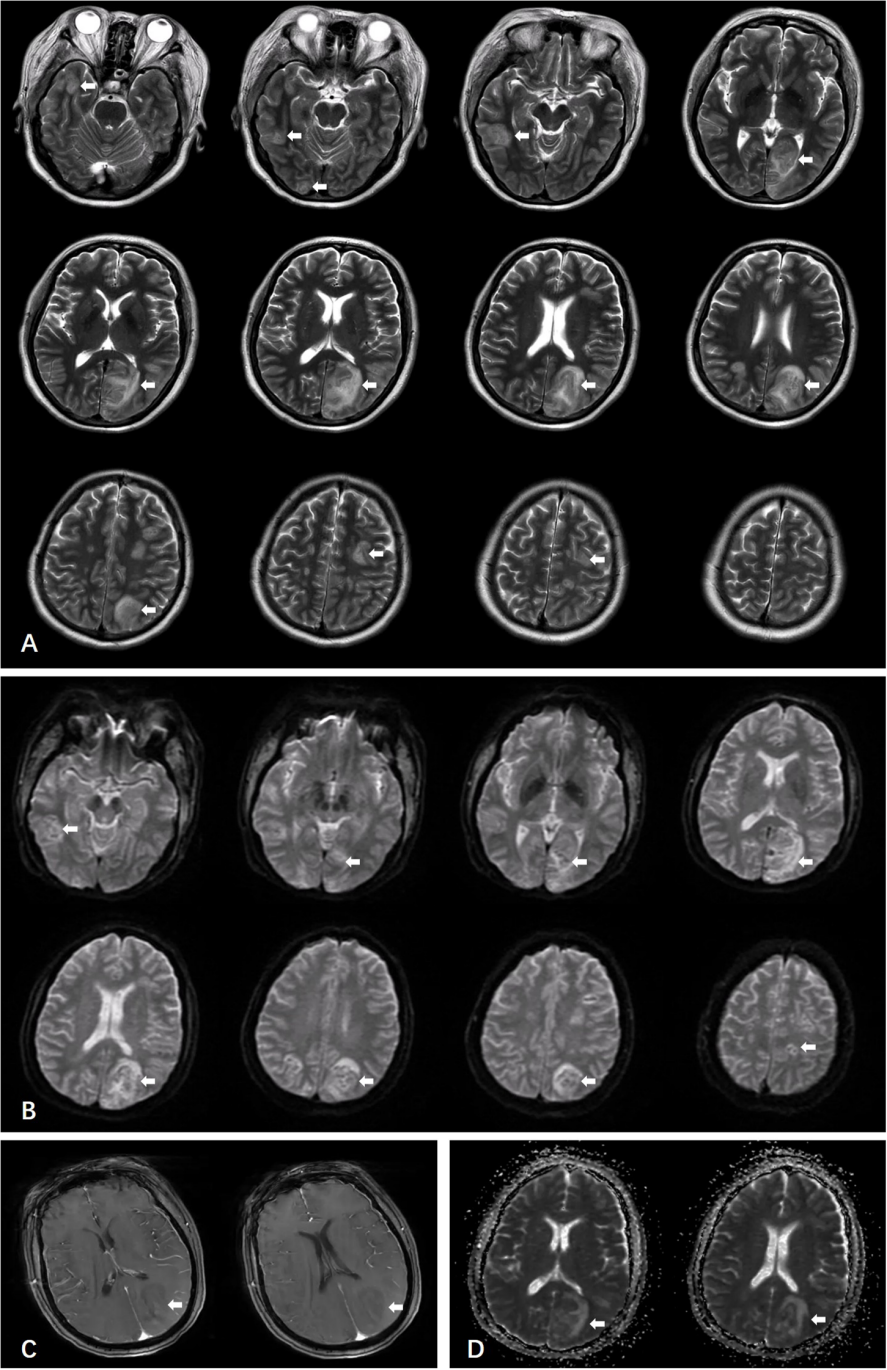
Multiple nodules and irregular patchy lesions showing long T1 and long T2 mixed signals are seen in the bilateral frontal lobes, parieto-occipital lobes, and centrum semiovale. The largest lesion, located in the left parieto-occipital lobe, measures approximately 5.0 × 2.8 cm (A, B). The lesions appear slightly hyperintense on DWI, with a small area of hypotintensity in the left occipital lobe (B). Post-contrast imaging shows heterogeneous mild enhancement of the lesions (C). Multiple hypointense areas are observed on the ADC map (D).The lesion is shown as the white arrow in the picture.
